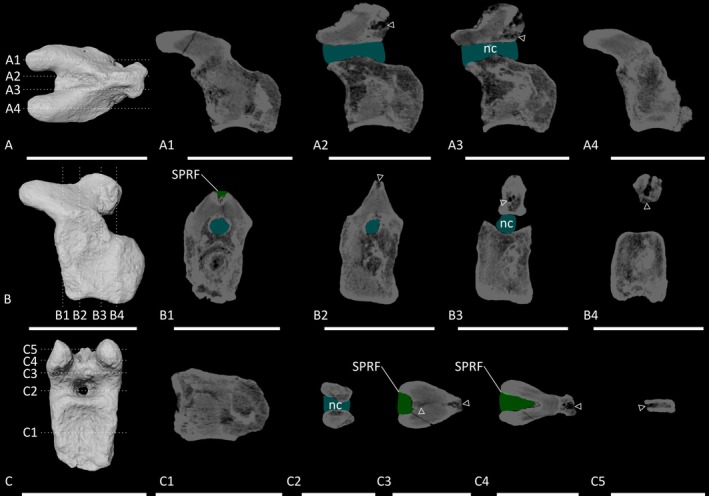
CT scan of *Ibirania parva* middle caudal vertebra MPMA 08–0060‐07. (A–C) 3D rendering (A) dorsal view, (B) left lateral view, (C) anterior view; (A1–A4) CT scan slices in parasagittal cross‐section, (B1–B4) CT scan slices in transverse cross‐section, (C1–C5) CT scan slices in horizontal cross‐section. nc, neural canal; SPRF, spinoprezygapophyseal fossa. Key: Blue highlight, neural canal; green highlight, neural spine fossa; white arrow, pneumatic bone texture. Scale bars = 100 mm.
